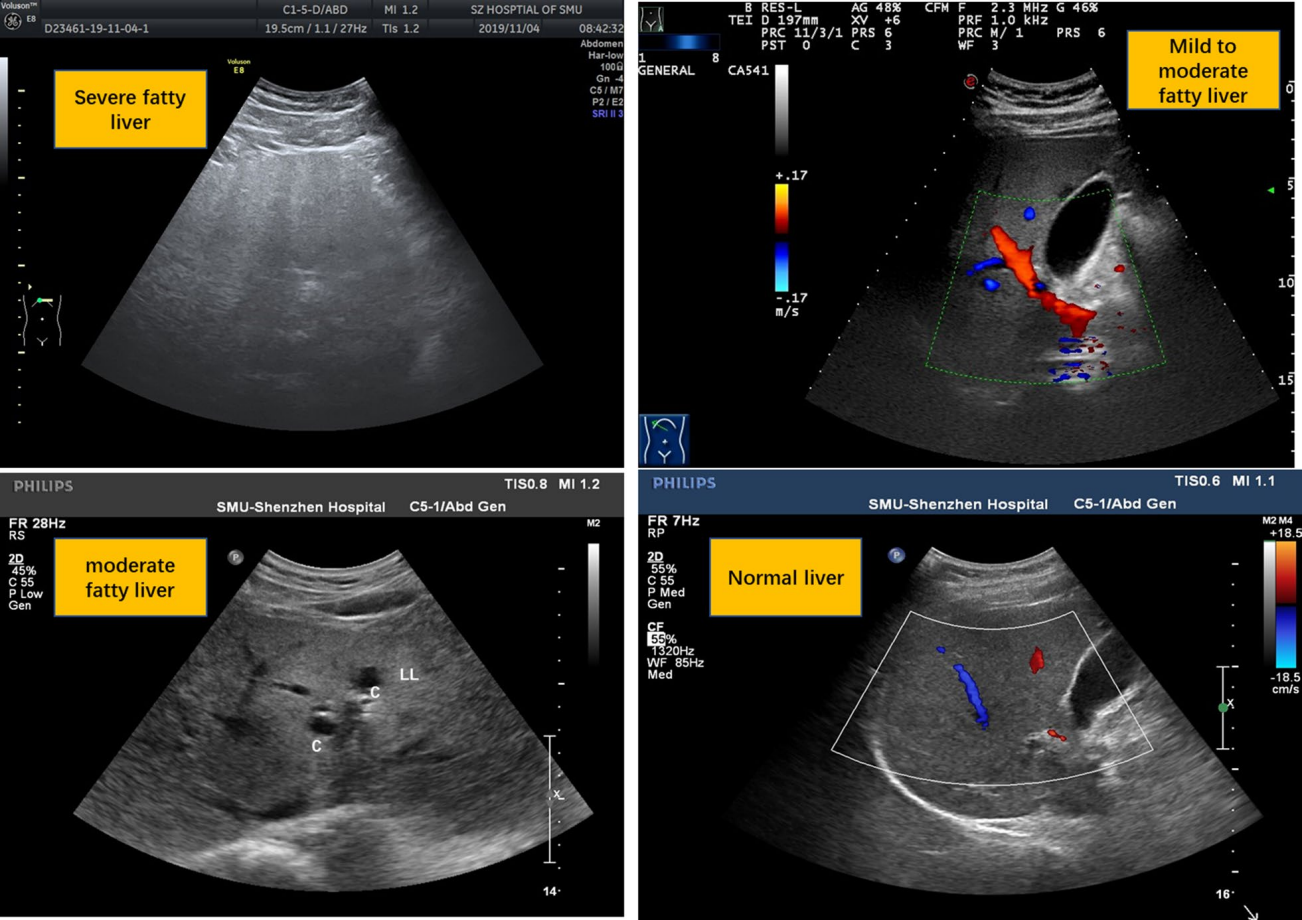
Ultrasonographic images of fatty liver at different degrees
